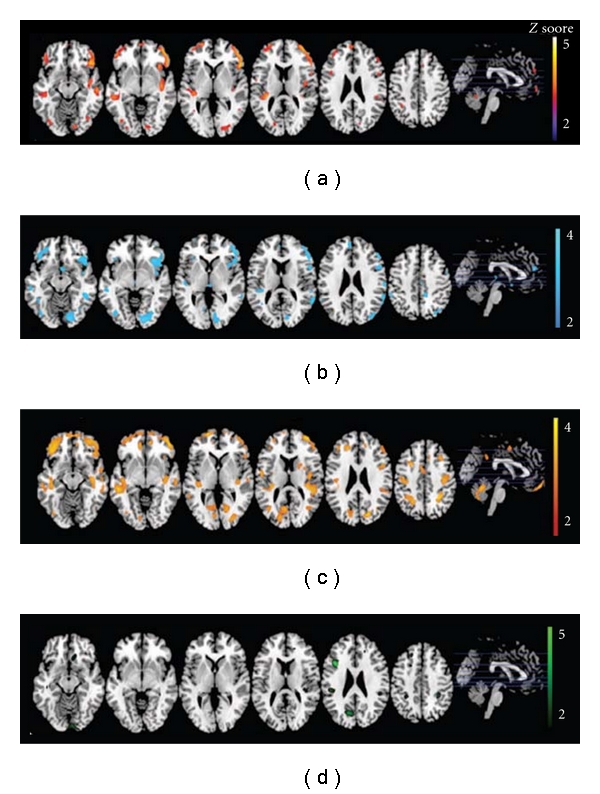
The first 2 rows display maps from subjects with a maternal family history of Alzheimer's disease (FHm) as compared with subjects with no family history (FH-) (a) and subjects with a paternal family history (FHp) (b). Row (c) shows gray matter volume (GMV) reductions in APOE *ε*4-negative FHm subjects compared with APOE *ε*4-negative FH subjects. Statistical parametric maps showing GMV reductions in normal FHp subjects as compared with FH subjects are in row (d). Areas of GMV decrease are represented on purple-to-yellow, blue-to-light blue, dark orange-to-yellow, and green-to-light green color-coded scales for the 4 contrasts, reflecting *Z* scores between 2 and 5 for the upper contrast and between 2 and 4 for the lower 3 contrasts. Areas of gray matter volume decrease are displayed on a standardized spatially normalized MRI (adapted with permission from [27]).
